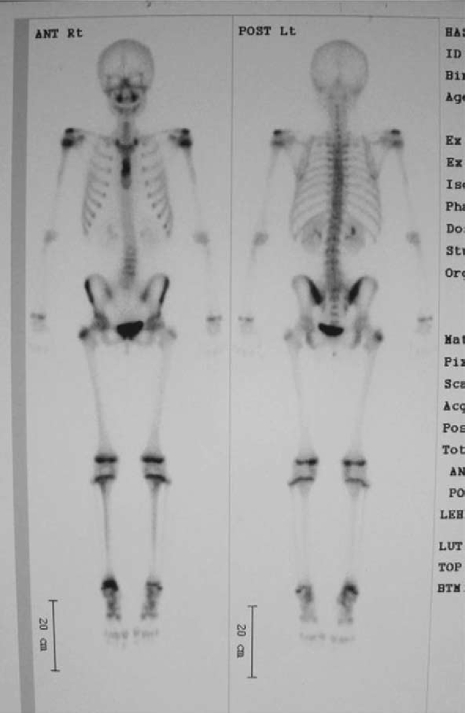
Technetium bone scintigraphy reveals increased uptakes in the sternum, the greater trochanter of the right femur, and the right distal tibia.
